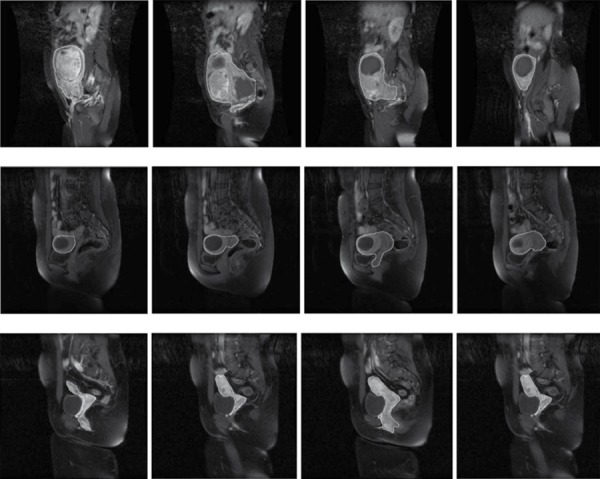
A 38-Year-Old Woman With Uterine Fibroid, Results of Automatic Segmentation of the Sagittal Images of MRI by Proposed Method
